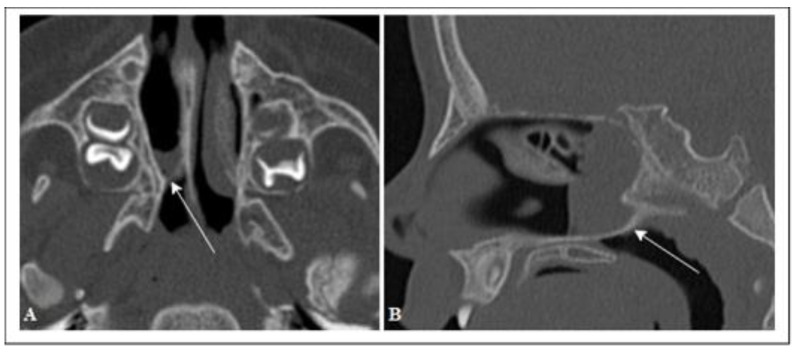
Axial CT scan (A), sagittal reconstruction (B) of a child with right unilateral choanal atresia. Mixed atresia wall; bone (arrows).
